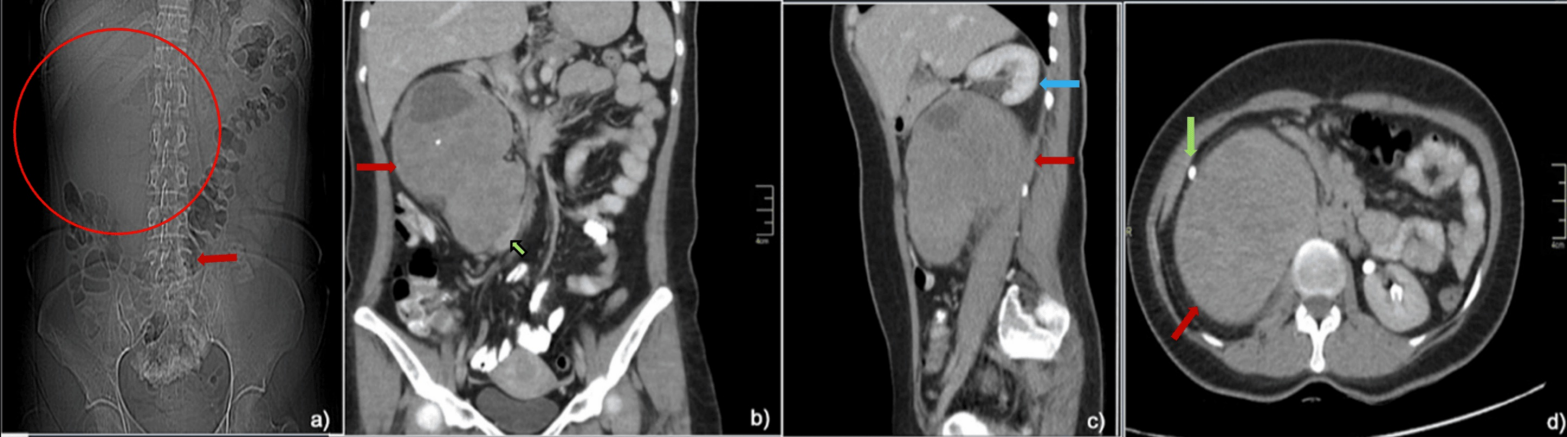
Contrast-enhanced abdominal CT (a) Localizer showing a space-occupying lesion in the right hypochondrium (outlined by a red circle) that displaces the hepatic flexure of the colon and the transverse colon toward the hypogastrium/mesogastrium (red arrow). (b) Right retroperitoneal mass (red arrow) with well-defined borders and heterogeneous density due to low-attenuation areas (which may correspond to areas with cystic degeneration) and a small calcification, causing medial displacement and compression of the inferior vena cava (green arrow). (c) Proximal and lateral displacement of the right kidney (blue arrow) caused by the retroperitoneal mass (red arrow). (d) Ipsilateral ureteral lateralization (green arrow). CT: computed tomography
